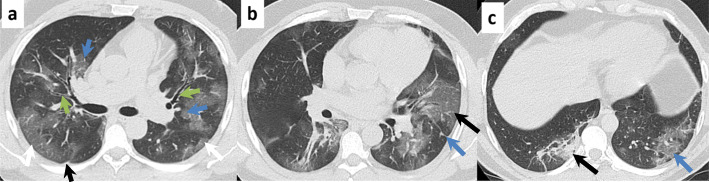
Sequence of images of a 79-year-old patient with fever, dyspnea, and myalgia of 10 days of evolution. The pulmonary parenchymal window thin section computed tomography shows the following: a Presence of ground glass opacities in the middle third of both hemithorax, of central distribution (blue arrows) and peripheral distribution (white arrows); parenchymal band declining in the upper third of the left lower lobe (gray arrow), a subpleural band (black arrow) and thickening of the axial peribronchovascular interstitium (green arrows) are added. b “Crazy cobblestone” pattern in the lobe of the lingula (black arrow) together with a pleural traction band (blue arrow). c Consolidation in the posterior segment of the right lower lobe associated with a parenchymal band in the same segment (black arrow)
